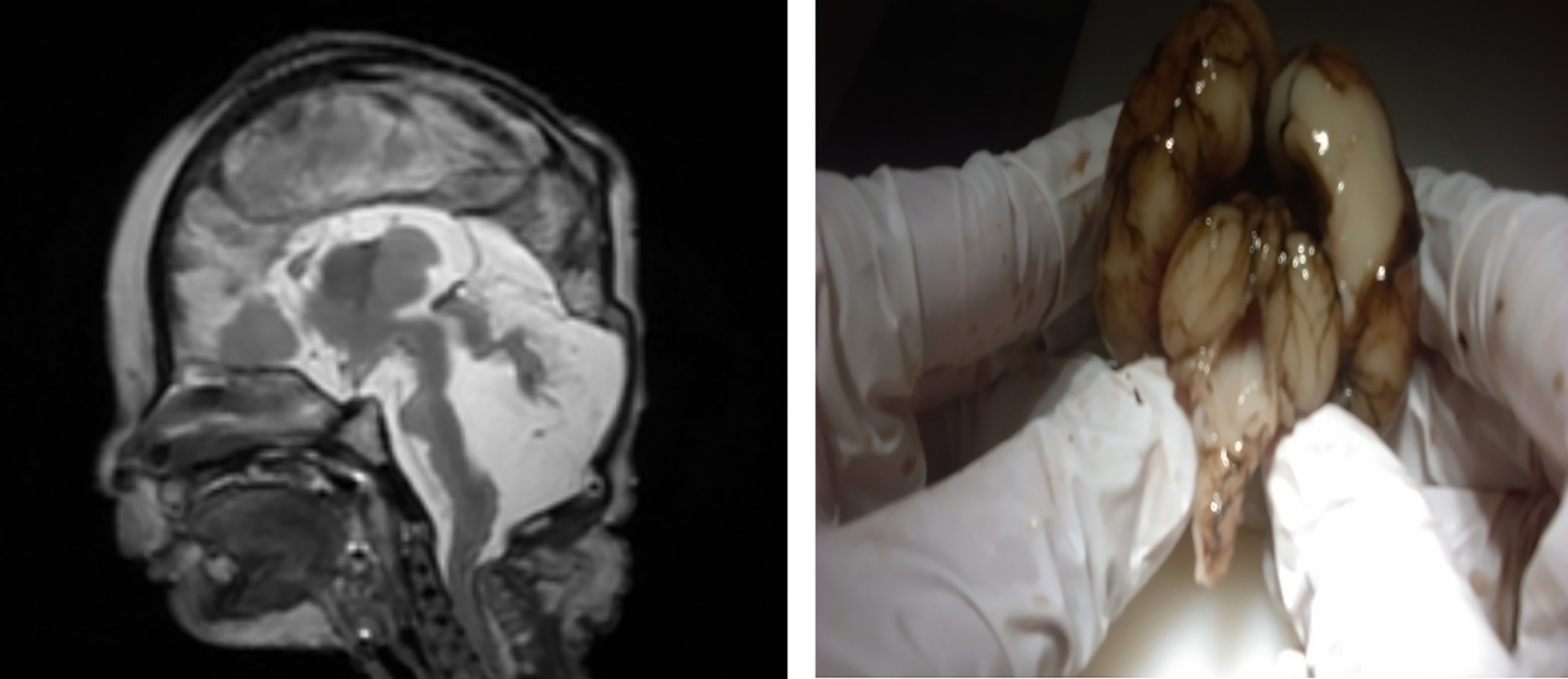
Comparison between post mortem MRI at 3T, T2 WI and conventional autopsy of a 22 weeks of gestation fetus with Dandy Walker malformation. The images depict enlarged posterior fossa, cystic dilatation of the fourth ventricle extending posteriorly, and vermis hypoplasia.
